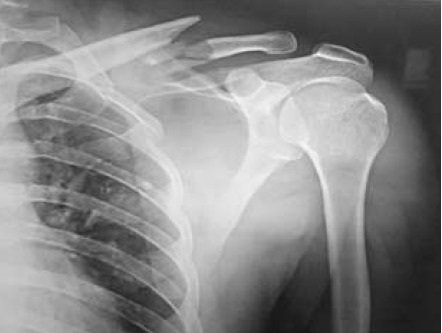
Fig. 4: Pre and Post op radiographs of left shoulder F4:
